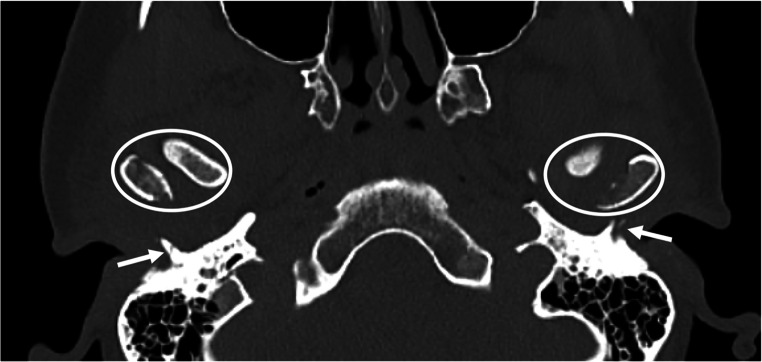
Computed tomography showing bilateral tympanal bone fracture (arrows) associated with bilateral fracture of mandibular condyle with internal luxation (circles). The victims fell on the ground with an impact on the chin
